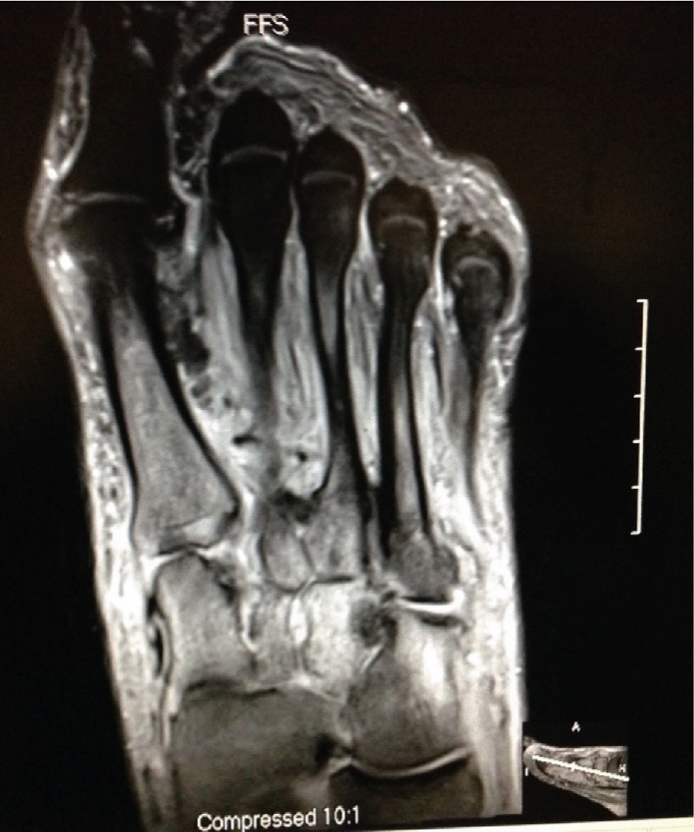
T2-weighted MRI of an example Charcot foot that demonstrates bone edema in a multitude of tarsal–metatarsal bones.
